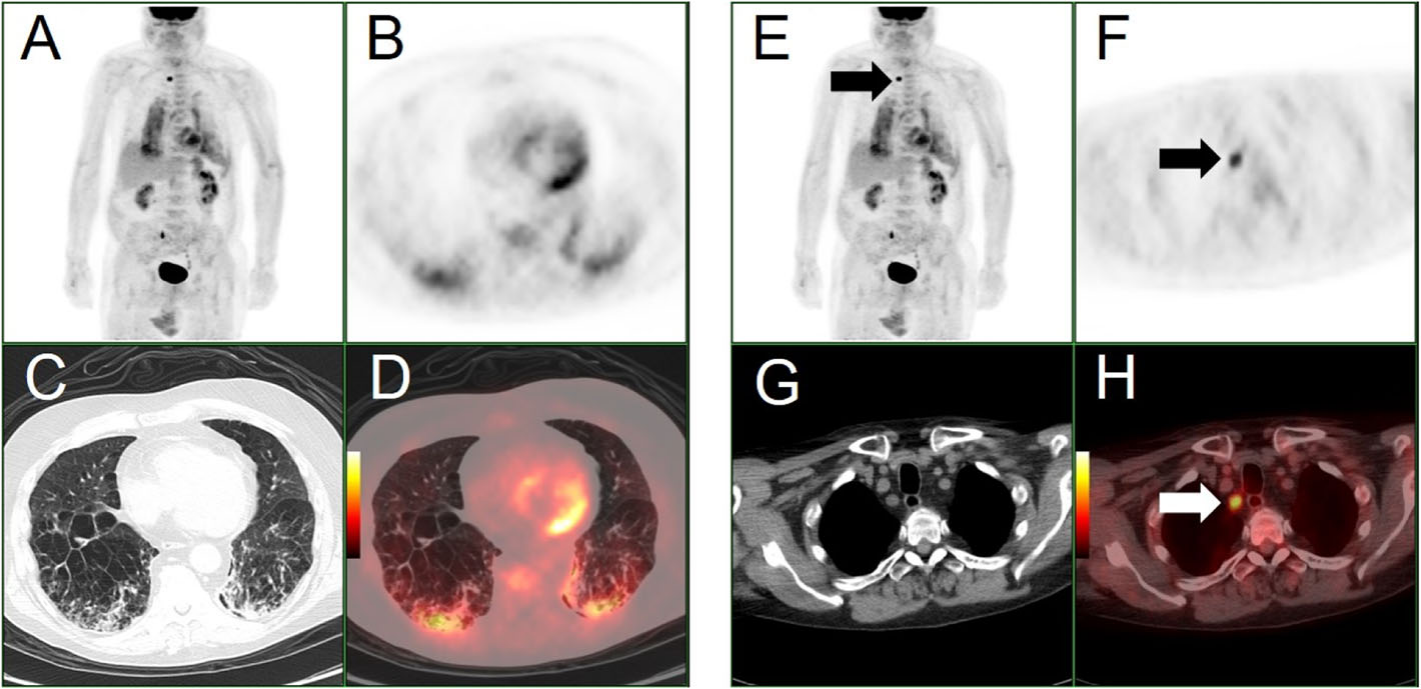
a PET maximum intensity projection (MIP), b–d: axial attenuation corrected PET, axial CT lung windows and axial fused PET/CT images through the lower thorax. e PET maximum intensity projection (MIP), f–h: axial attenuation corrected PET, axial CT mediastinal windows and axial fused PET/CT images through the superior mediastinum. These images highlight intense bilateral parenchymal metabolic activity, more prominently in the right lower lobe (images a–d) and high uptake in an ATS station 2R lymph node (e–h). Please note spurious right ureteric activity on the MIP images (images a and e)
